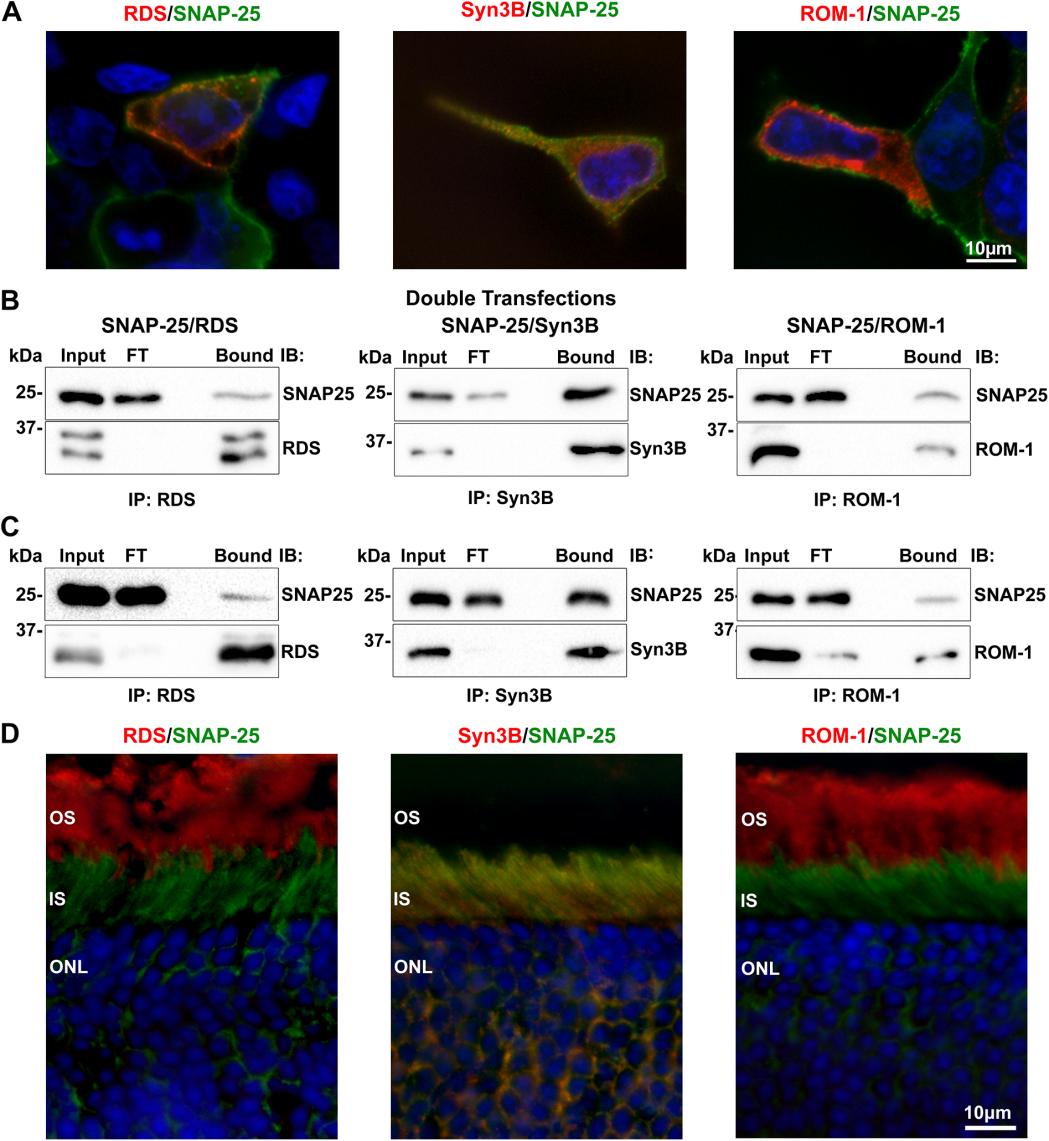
RDS and ROM-1 interact directly with SNAP-25 *in vitro* and *in vivo*. A. HEK293 cells were co-transfected with SNAP-25 (green) and either RDS (RDS-CT, red), ROM-1 (ROM1-CT, red) or Syn3B (Syn3B-529, red) antibodies. B. CHAPS-solubilized cell extracts underwent co-IP for RDS (RDS-CT), Syn3B (mAB 12E5), and ROM-1 (mAB 2H5) antibodies. Resulting blots were probed for SNAP-25, RDS (mAB 2B7), Syn3B (mAB 12E5), or ROM-1 (mAB 2H5) antibodies. C. CHAPS-solubilized WT retinal extract underwent IP and reducing SDS-PAGE/WB with the same antibodies as in B. D. WT retinal sections underwent IF to study distribution of SNAP-25 (green) in the outer retina in relation to labeling with RDS (RDS-CT, red), Syn3B (Syn3B-529, red), or ROM-1 (ROM1-CT, red) antibodies. Scale bars: 10 μm. OS: outer segment, IS: inner segment, ONL: outer nuclear layer. FT: flow through.
